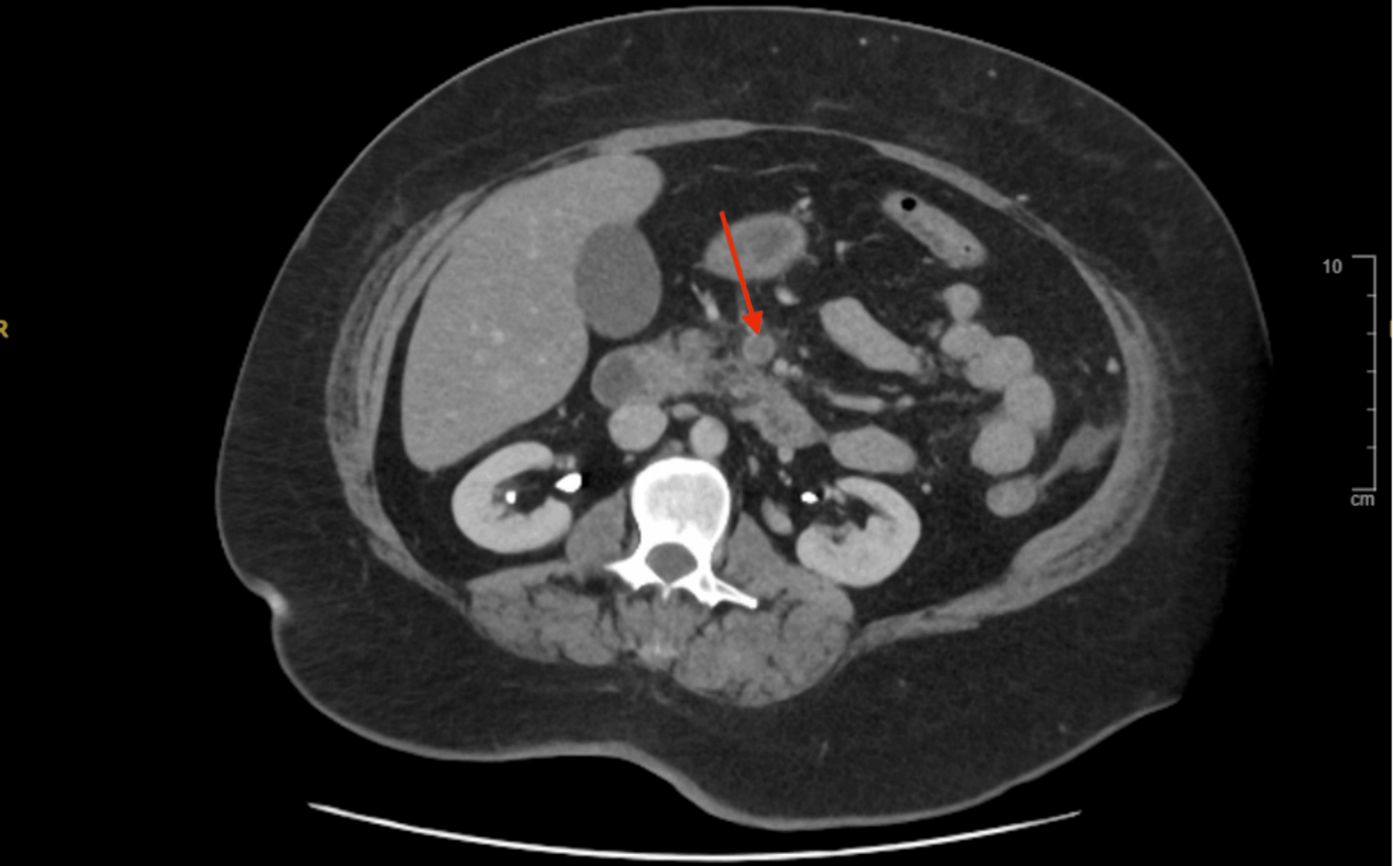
Axial contrast-enhanced CT scan showing acute superior mesenteric vein thrombosis (red arrow)
